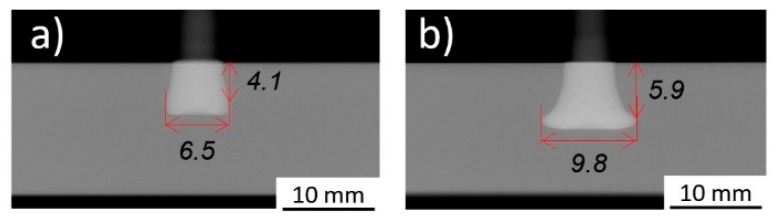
X-ray tomography of: (a) Condition 33 (RS = 19,000 rpm; FT = 1.8 s; FoT = 1.5 s; FF = 1500 N; FoF = 4500 N); (b) Condition 36 (RS = 19,000 rpm; FT = 1.8 s; FoT = 1.5 s; FF = 2500 N; FoF = 5700 N).
